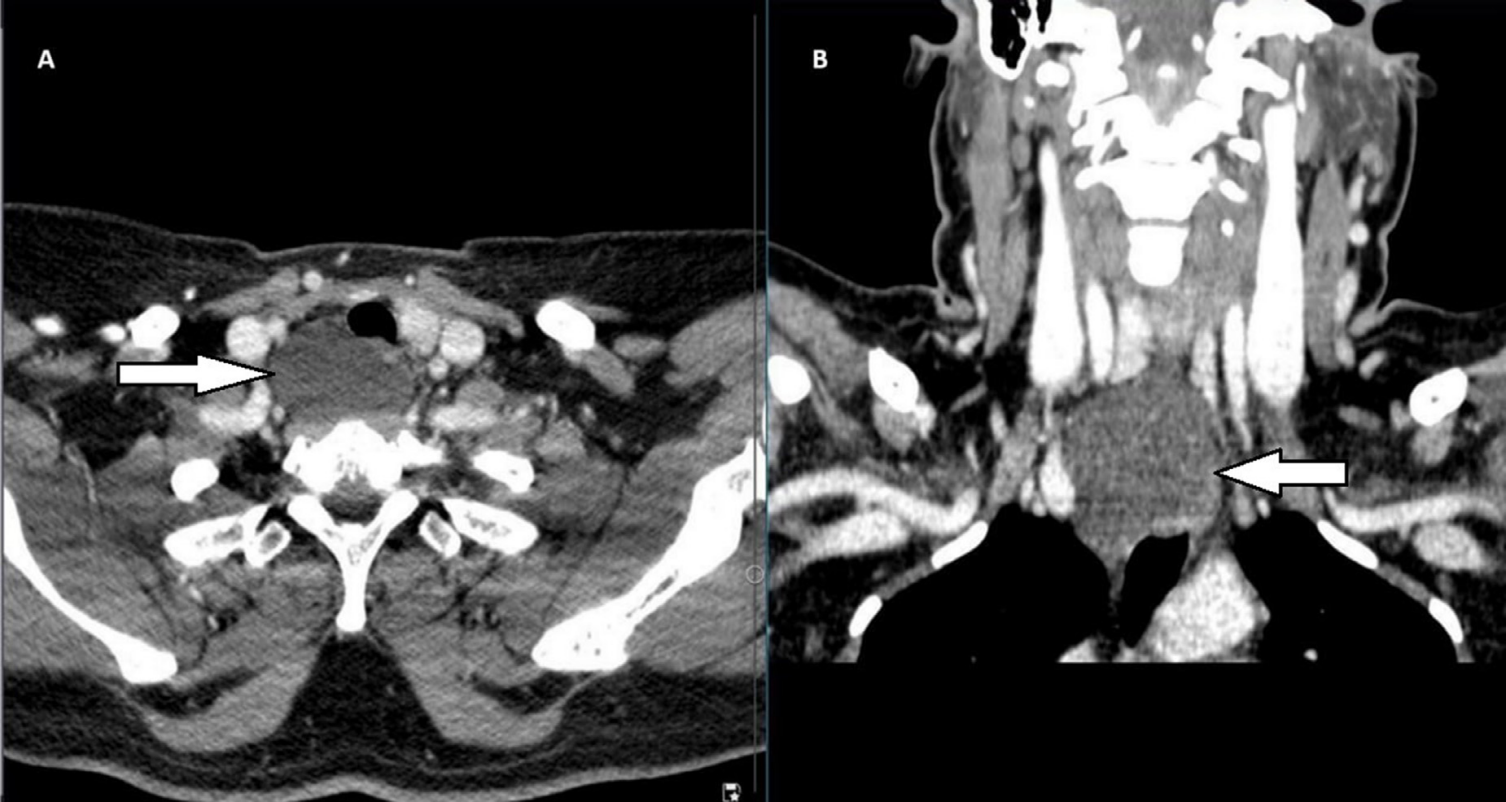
CT scan of neck and upper chest with contrast (axial and coronal section), shows well defined thin wall cystic lesion (white arrow), located in right side posteriolateral to trachea and extending to the mediastinum, causing mild surrounding mass effect, with no enhancement.
